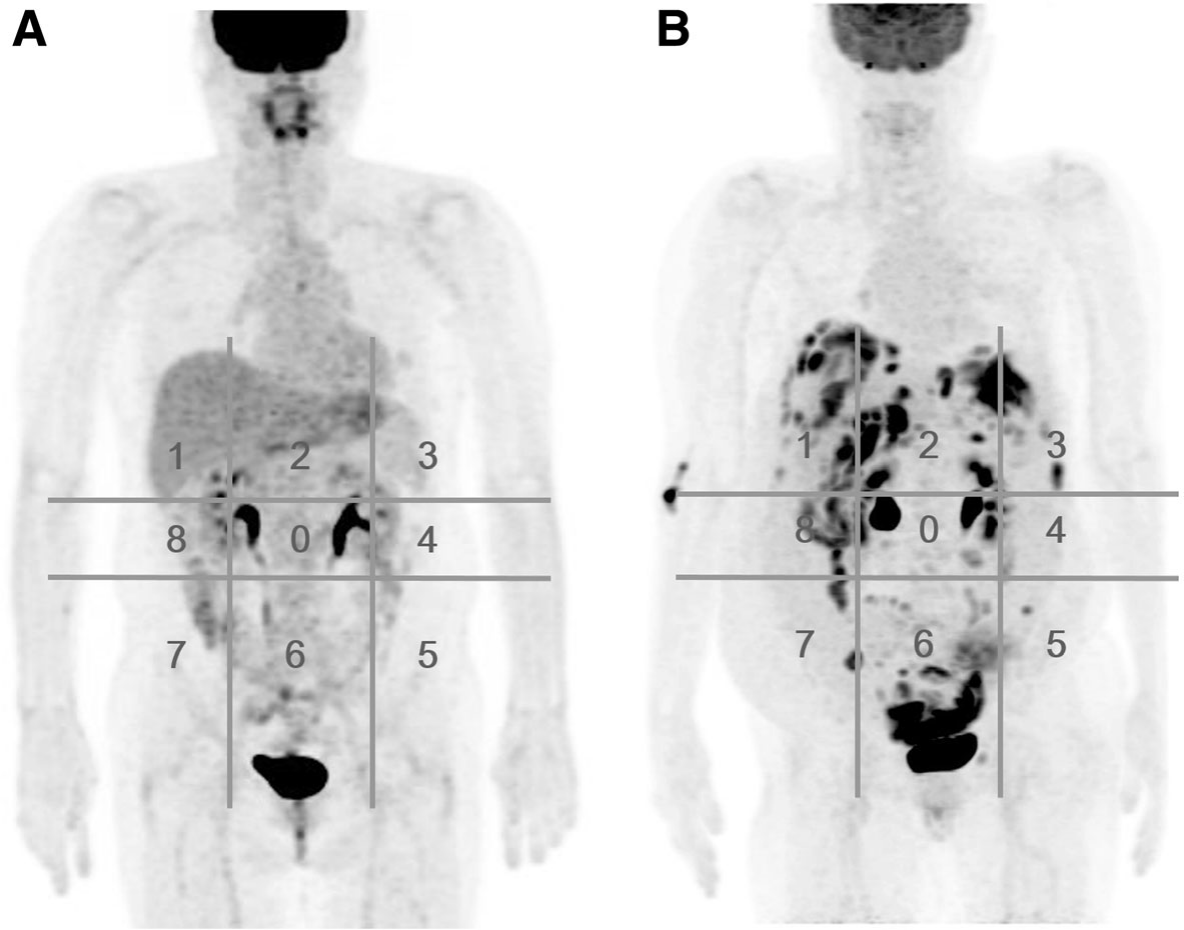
Representative F-18 FDG PET/CT images. F-18 FDG PET/CT images were analyzed according to 9 regions covering the whole peritoneal cavity including pelvis. Each quadrant was considered as a possible implant site and was evaluated separately. The regions were numbered as follows; 0, central; 1, right upper; 2, epigastrium; 3, left upper; 4, left flank; 5, lower left; 6, pelvis; 7, right lower; and 8, right flank. a F-18 FDG PET/CT images represents no evidence of peritoneal carcinomatosis in patients with ovary cancer. b F-18 FDG PET/CT shows ovary cancer patients with peritoneal carcinomatosis
